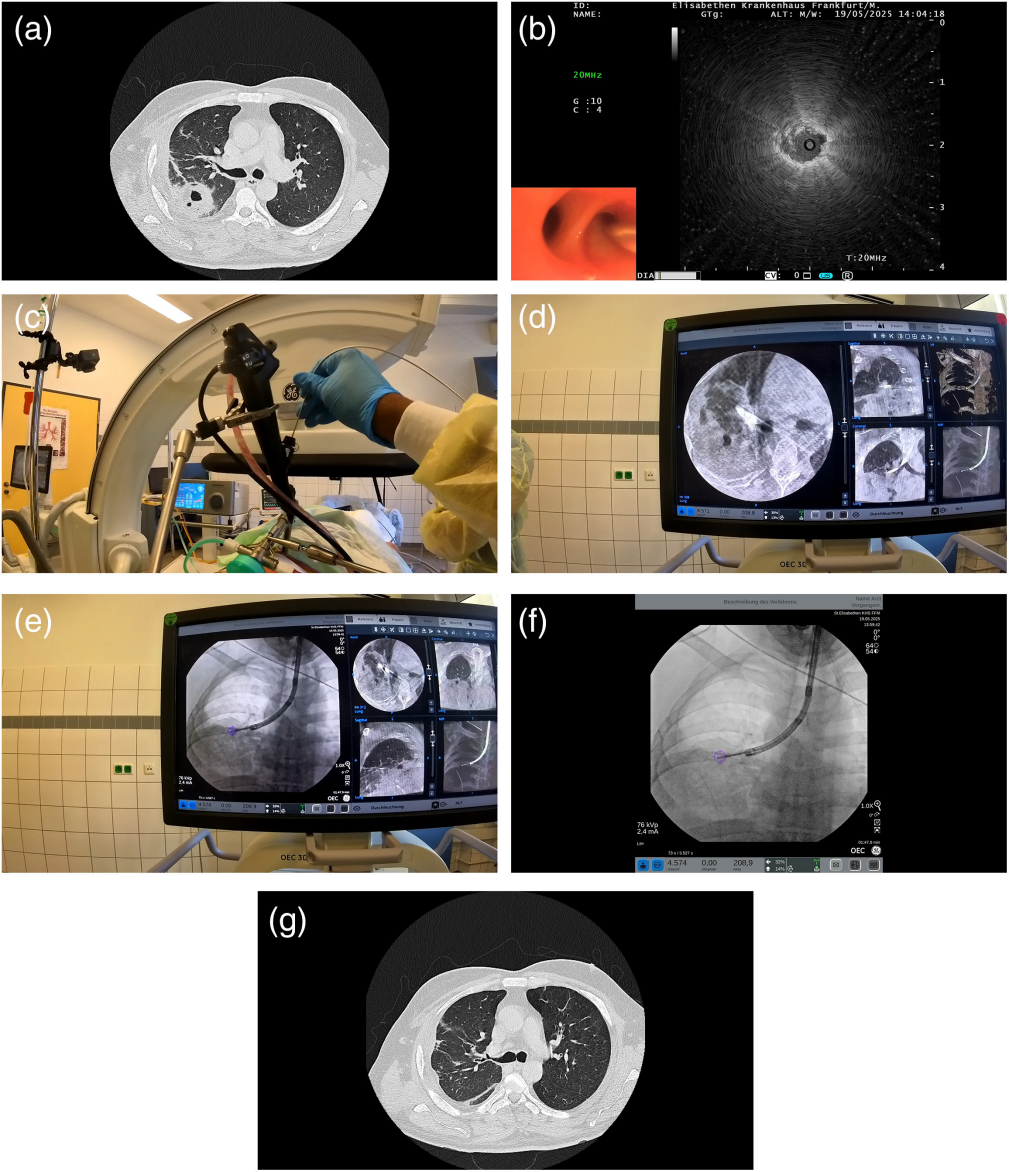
(a) Initial CT‐scan showing lung abscess in right upper lobe. (b) Positive rEBUS signal after locating upper lobe airway leading to lung abscess. (c) Aspiration needle being advanced into docked bronchoscope. (d) CBCT‐Imaging showing needle placement within lung abscess. (e, f) Aspiration of lung abscess under augmented fluoroscopy. (g) Follow‐up CT scan showing resolution of the upper lobe abscess.
